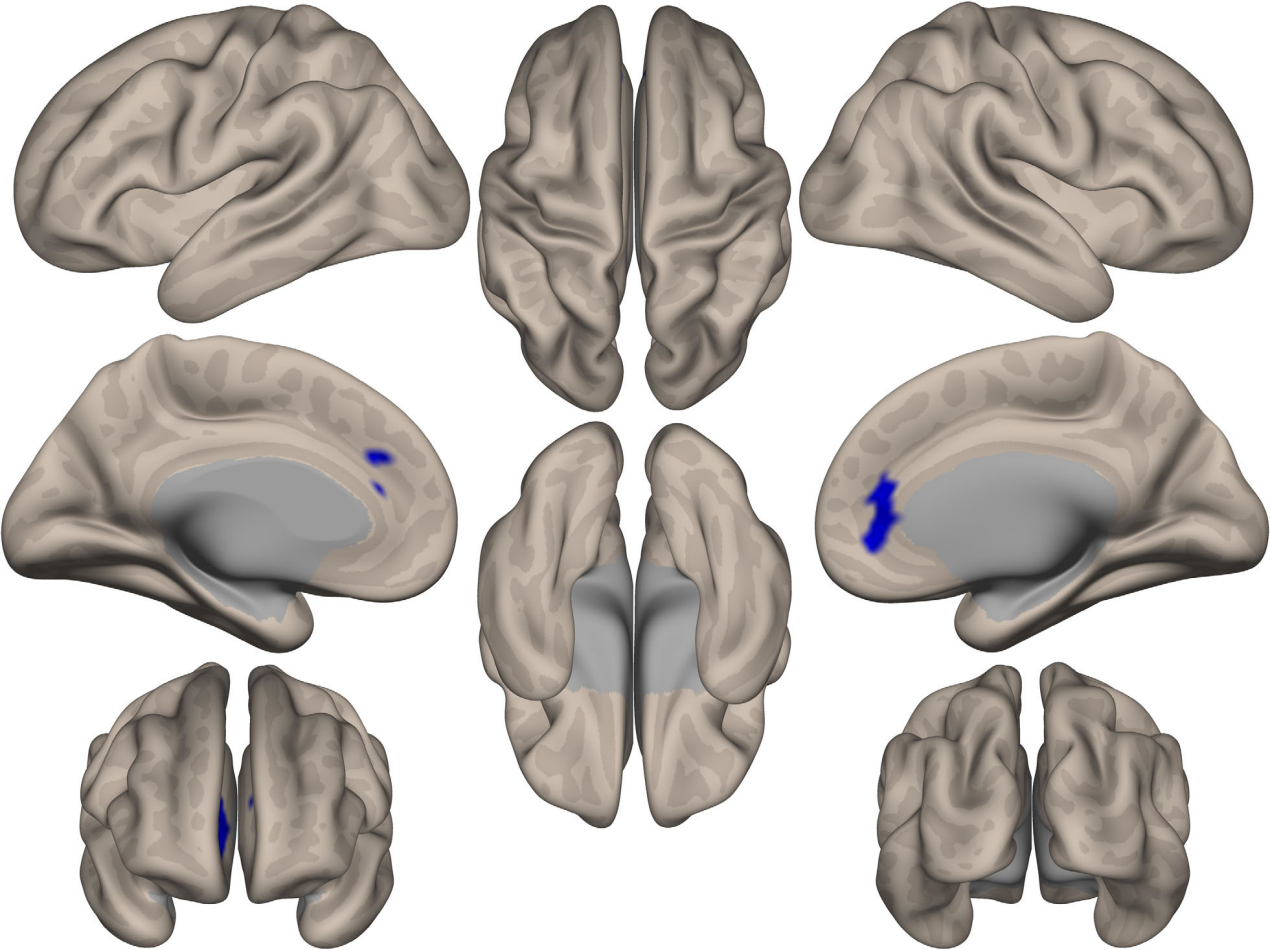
Decreased degree centrality associated with P_αβ_ in patients with amyotrophic lateral sclerosis (ALS). Analysis of resting‐state functional magnetic resonance imaging using intrinsic connectivity contrast demonstrated that the P_αβ_ was associated with decreased degree centrality at the medial prefrontal cortex. This area consisted of the anterior cingulate gyrus and frontal pole in patients with ALS. The results were assessed using a cluster‐forming height threshold of *P* < 0.01 and family‐wise error‐corrected cluster‐size threshold of *P* < 0.05.
